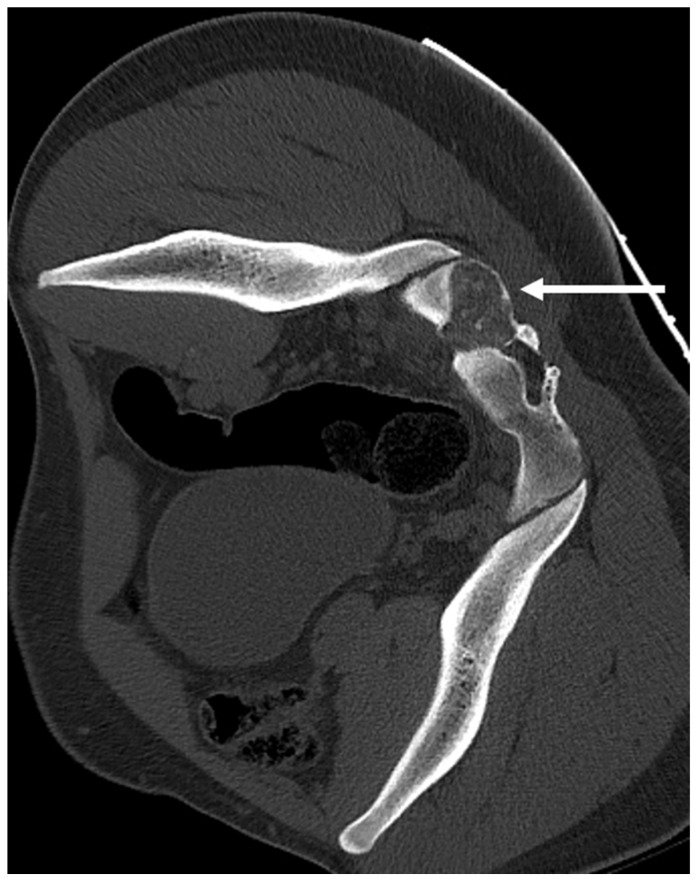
Axial CT image of an osteoblastoma of the sacrum (white arrow). Note the typical lytic and slightly expansile appearance and intralesional calcification.
